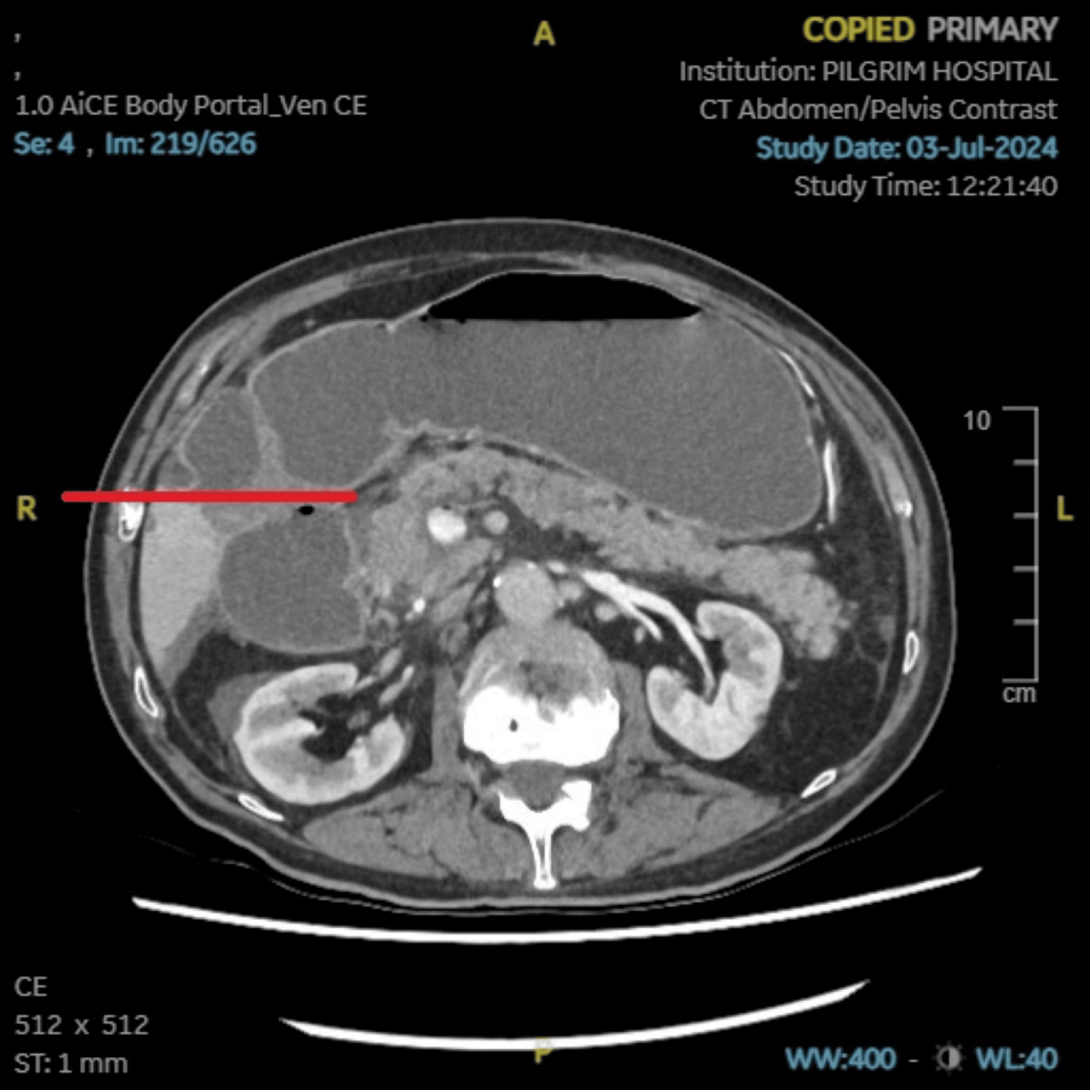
CT abdomen pelvis with contrast. Pancreas appears normal in size with subtle ill-defined hypodensity in the neck region associated with peripancreatic fluid extending to the superior recess of the lesser sac (pointed with red line).
